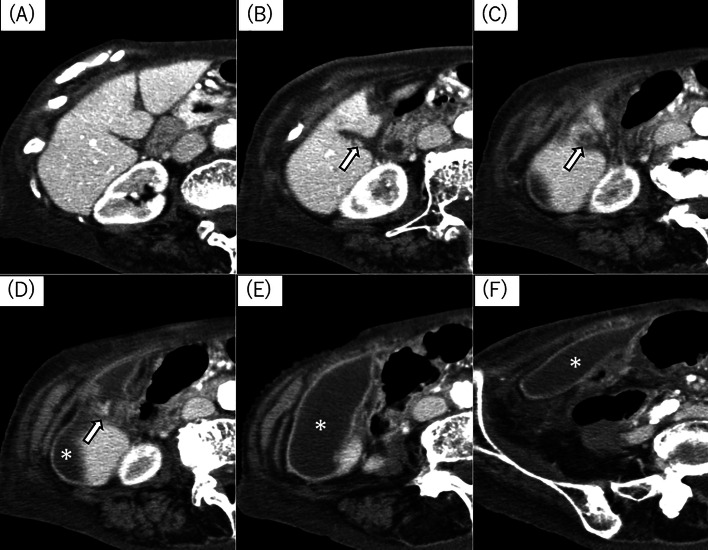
Abdominal computed tomography (CT) (axial image, A to F is arranged from the head to tail, respectively). Contrast-enhanced CT scan revealed an encapsulated fluid collection of >10 cm with a wall structure extending from the right upper quadrant to the right lower abdomen. The normal gallbladder was not visualized. A whirl sign observed in the CT indicates the torsion at the gallbladder neck (Fig. 1, arrow), with the body and base of the gallbladder being completely unidentifiable and appearing continuous with the encapsulated fluid collection (Fig. 1,*).
